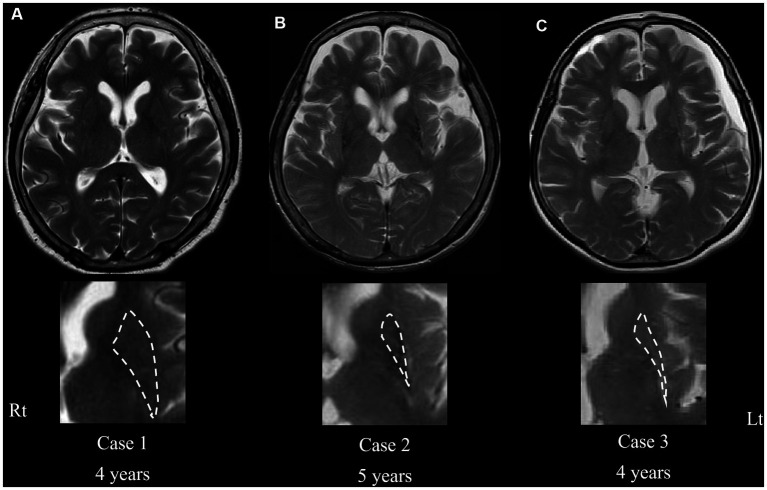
Transaxial T2-weighted magnetic resonance imaging of cases 1 (current case) (A), 2 (B), and 3 (C) at the level of the putamen. Severe atrophy is evident in cases 2 and 3 (B,C), whereas no atrophy in the putamen is shown in the current case (A), even in the advanced phase of the disease. Magnified images of the putamen are shown in the bottom column. Dashed lines indicate the border rims of the putamen. The “years” represents the periods from onset.
